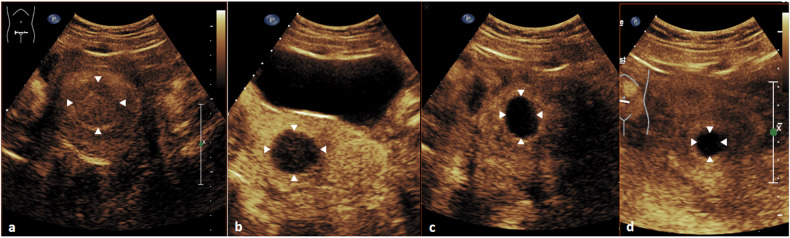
Contrast-enhanced ultrasound images of the uterus of a 41-year-old premenopausal woman with symptomatic uterine fibroids are shown. The predominant target fibroid in the fundus of the uterus was treated with USgHIFU. a Predominant target fibroid in the fundus of the uterus (white arrows) with contrast enhancement prior to HIFU treatment. b Immediately after HIFU ablation (2 h post-HIFU), a large area without contrast enhancement is shown (white arrows). c, d A significant reduction in fibroid volume of approximately 54% after 3 months (c) and 85% after 6 months (d) compared to the initial lesion volume was observed
